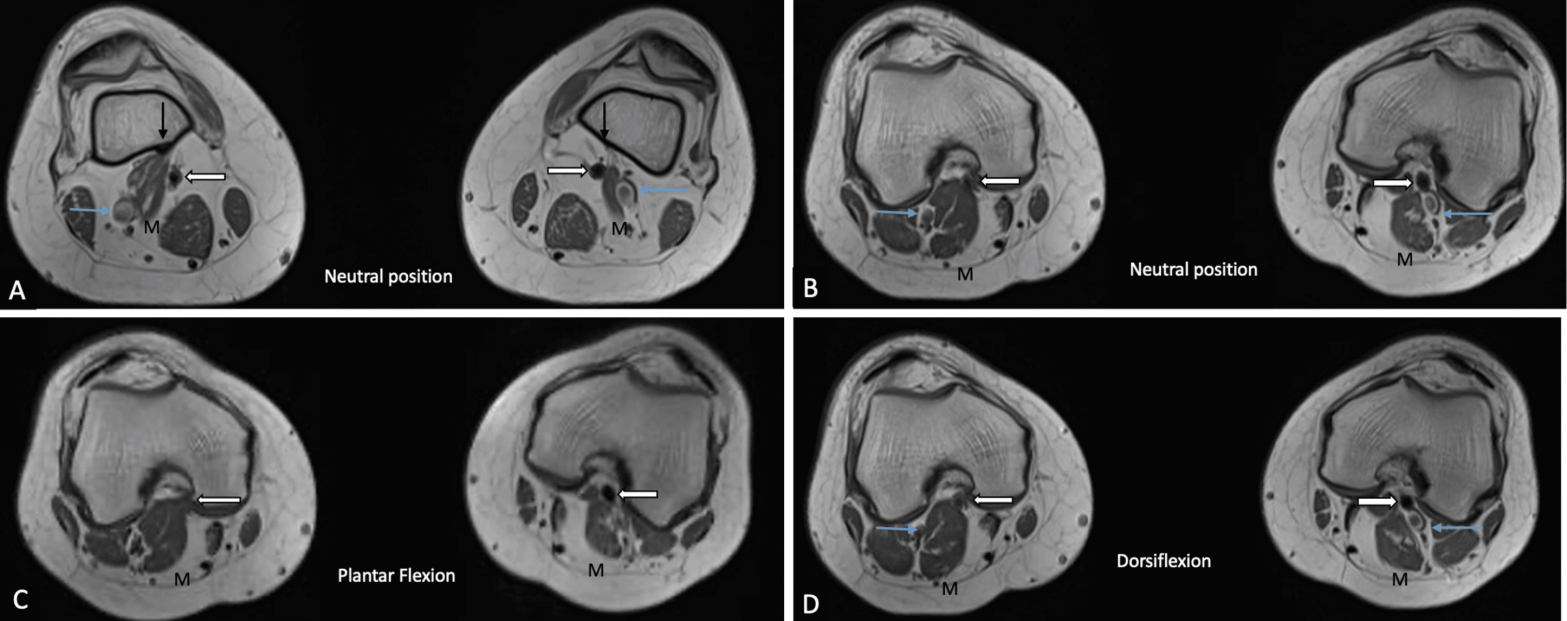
Axial T1-weighted images of the popliteal region in different dynamic maneuvers: 1A and 1B show the neutral position, 1C shows plantar flexion, and 1D shows dorsiflexion. Figure 1A shows an abnormally high and lateral insertion of the medial head of the gastrocnemius (M) just above the lateral aspect of the medial femoral condyle bilaterally (straight black arrow). The muscle belly is seen laterally deviated between the PV (blue arrow) laterally and PA (white arrow) medially, causing significant extrinsic compression of the PA on the right side (white arrow; Figure 1A-1B). On plantar flexion (Figure 1C), there was significantly increased compression of the PA (white arrow) on the right side, and no significant luminal narrowing of the PA (white arrow) was seen on dorsiflexion (Figure 1D). In addition, no significant compression of the left PA (white arrow) was seen on either plantar flexion or dorsiflexion (Figure 1C-1D). PV: popliteal vein; PA: popliteal artery
